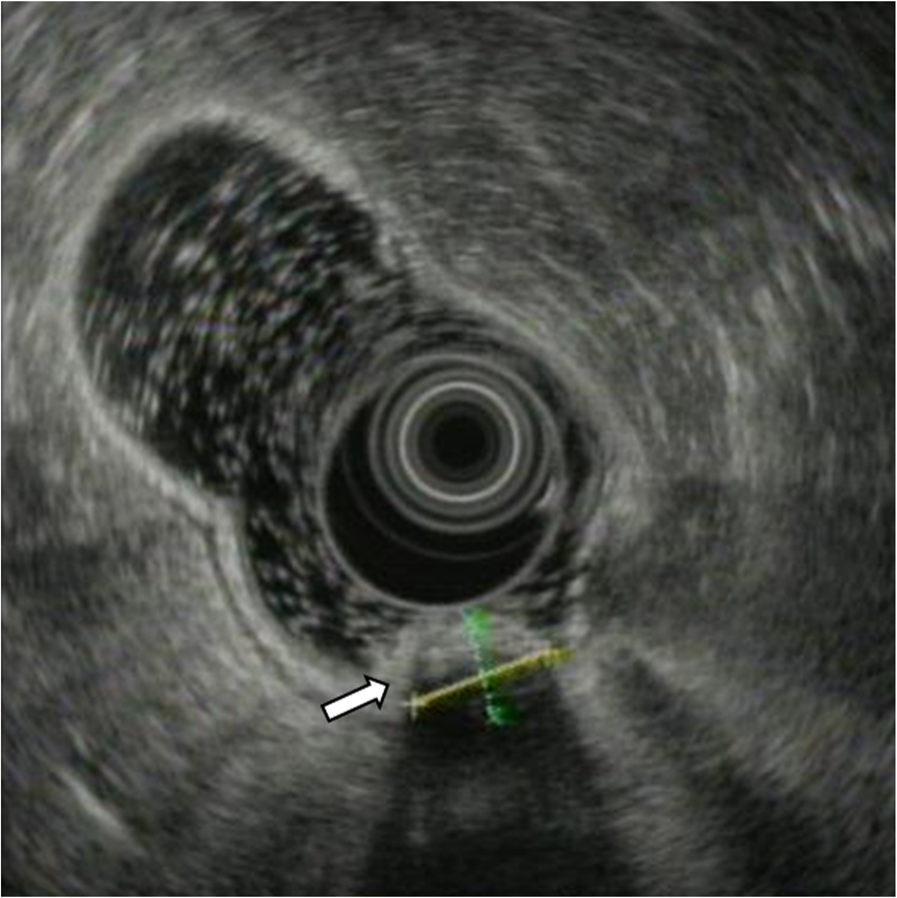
Endoscopic ultrasonographic finding shows a well-demarcated submucosal mass (arrow).
